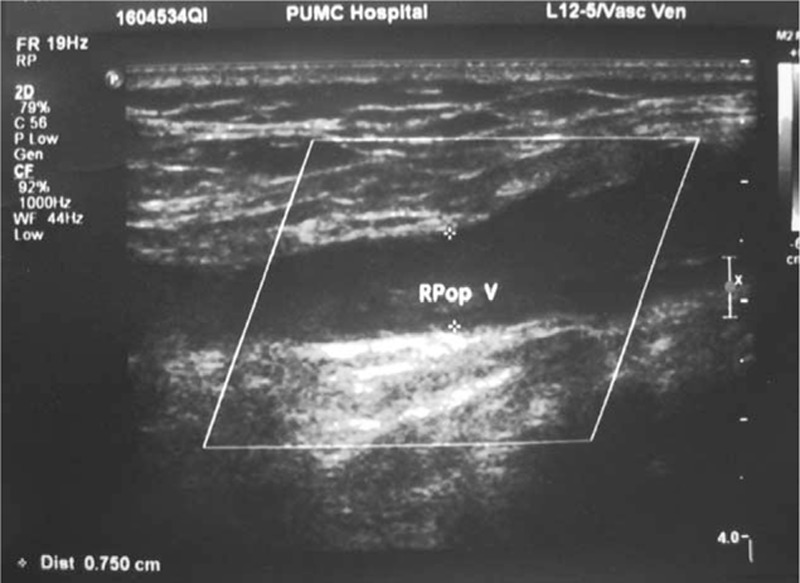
Lower limbs ultrasonography examination revealed thrombosis in the right popliteal vein in case 1.
